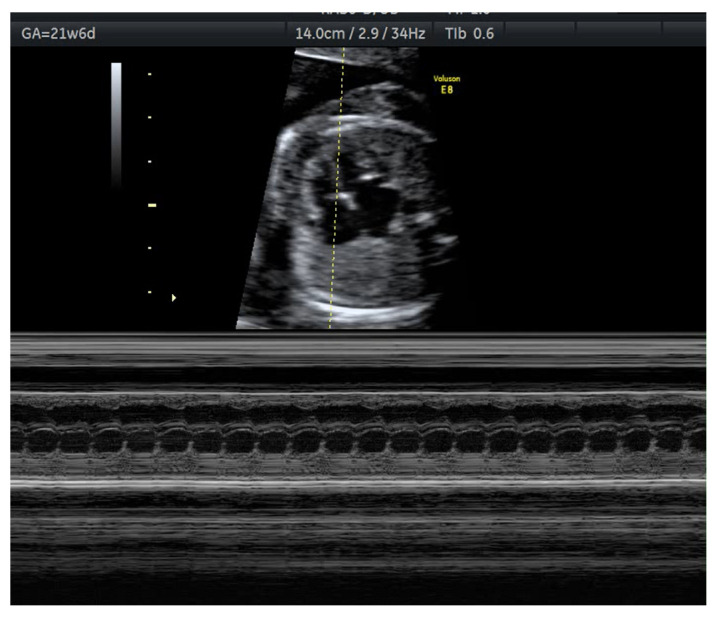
Foetal ultrasound M mode-foetal complete heart block in a pregnancy of 21 weeks of a mother with anti-Ro antibodies.
